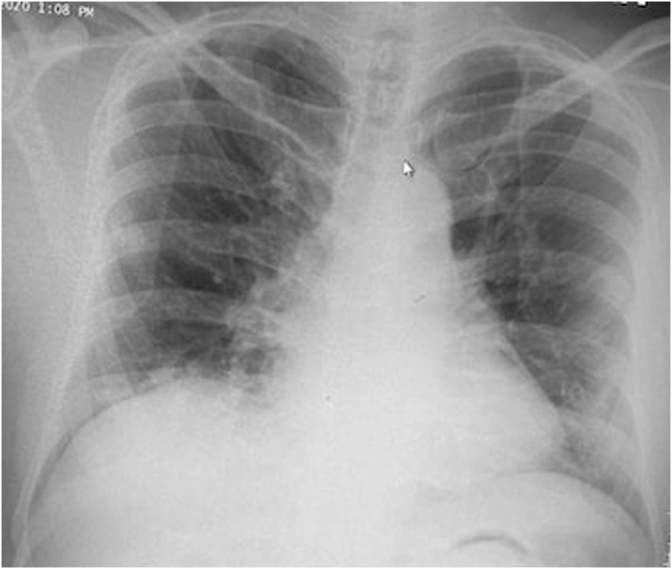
Plain chest X-ray demonstrated consolidation in the basal right lung.
